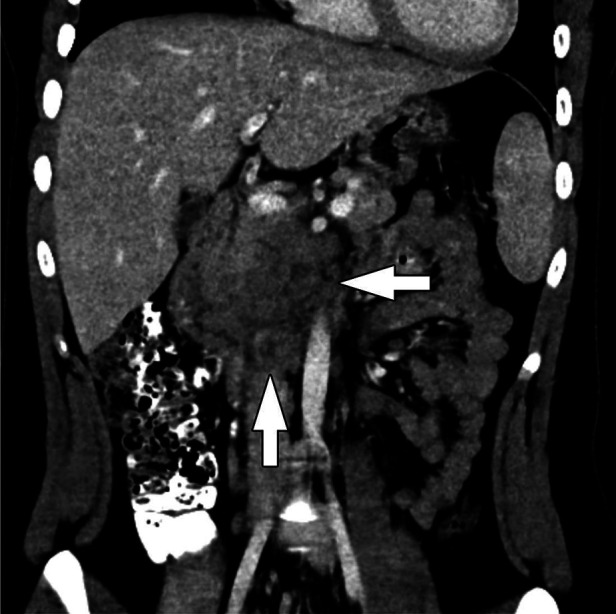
Acinar cell carcinoma. Coronal CT of the abdomen obtained in the portal venous phase following intravenous contrast material administration shows an infiltrative, hypoenhancing mass involving the head and uncinate process of the pancreas with adjacent conglomerate pathologic lymph node enlargement (arrows).
